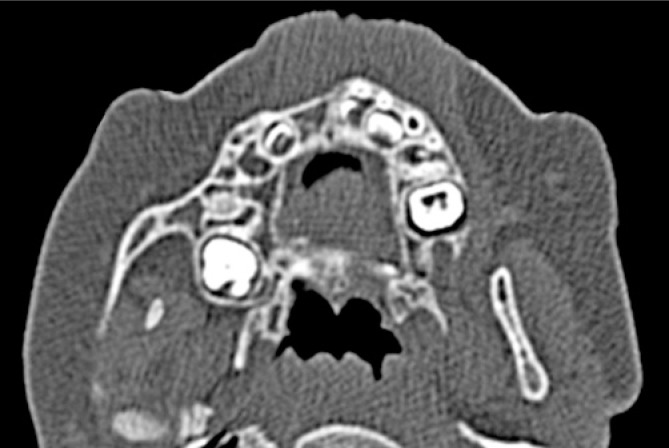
Axial computed tomography scan showing bone neoformation in the maxilla and no evidence of tumor recurrence
